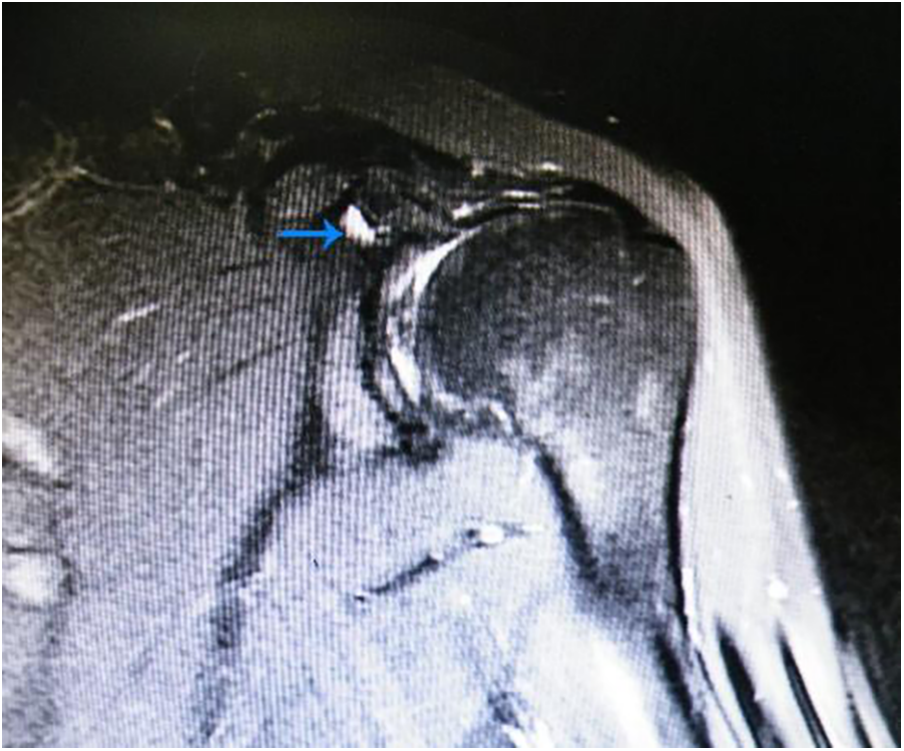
Preoperative radiographic images. The blue arrows indicate the type II superior labrum anterior and posterior (SLAP) lesion.
